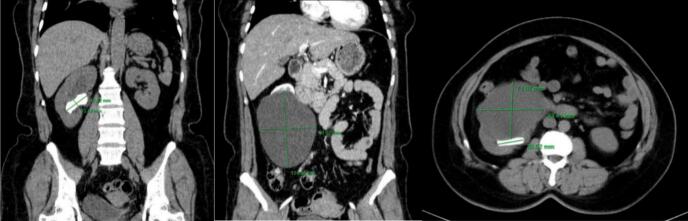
Image of the right simple renal cyst and ipsilateral renal calculi on CT of patient 1.
